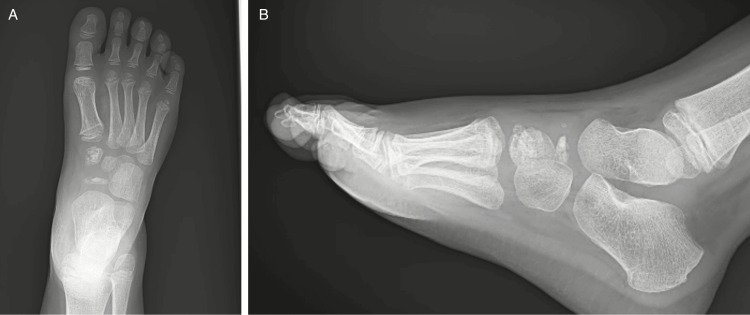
Radiographs from Patient 1’s initial visit, including anteroposterior (A) and lateral (B) views of the right foot of the five-year-old patient; note the “wafer-thin” (i.e., thin, sclerotic) appearance of the navicular, typical of Kohler disease, and the inconsistently fragmented appearance of the medial cuneiform.
